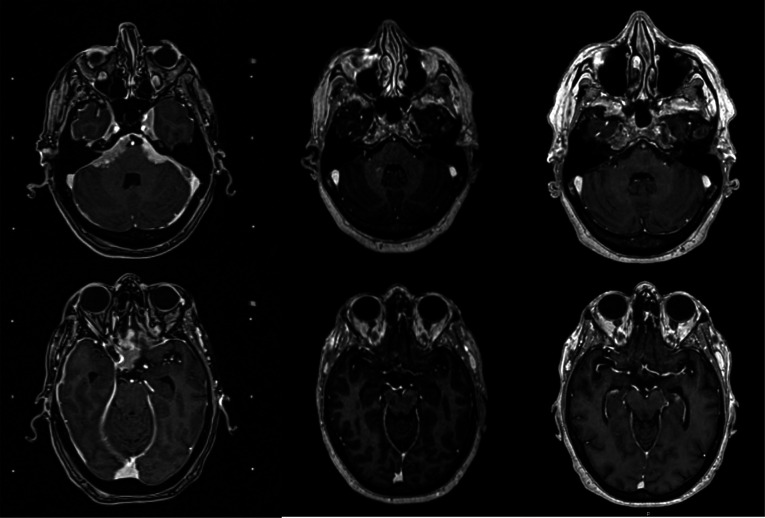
Contrast-enhanced T1 MR images at the time of radiosurgery (left column), at 6 months follow-up (middle column) and at 52 months follow-up (right column).
